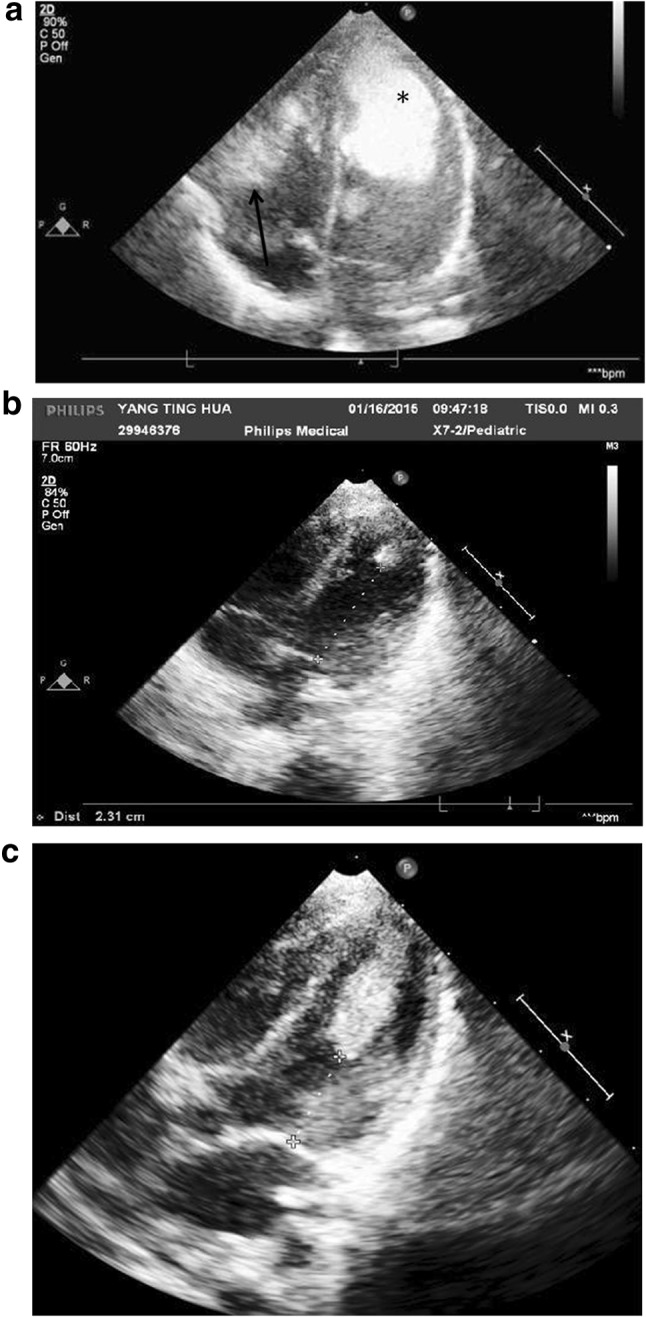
Case 1: a Echocardiogram of the first day of life. The LV tumor *Asterisk* occupied its apical half. The *arrow* indicates another small tumor in the RV free wall. b After 3 months of everolimus therapy, the LV tumor has regressed to a small nodule in the apex (*arrow*). c Two months after everolimus cessation, the tumor significantly rebounded; however, the LV chamber has grown to a satisfactory size
